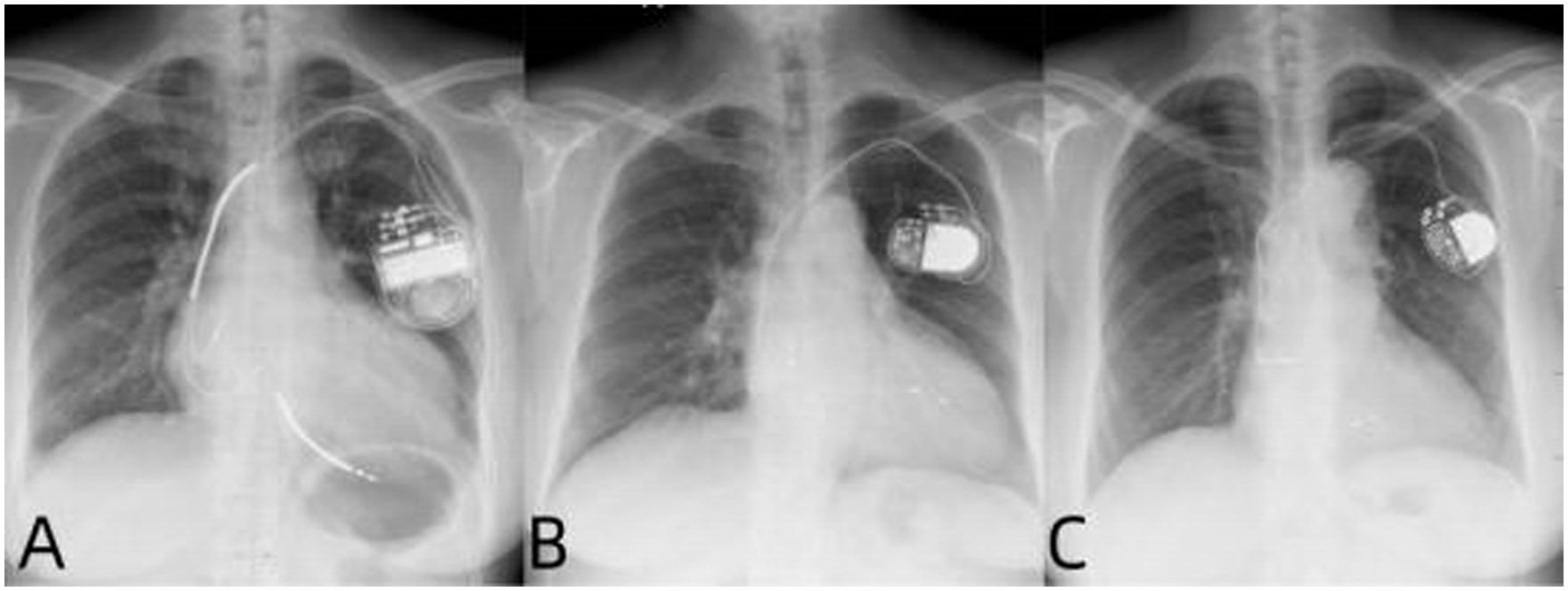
Postoperative x-ray images of three types of pacemaker mplantations. (A–C) Are the x-ray images after the implantation of BVP, LUVP-SVP, and LUVP-LBBAP respectively.
